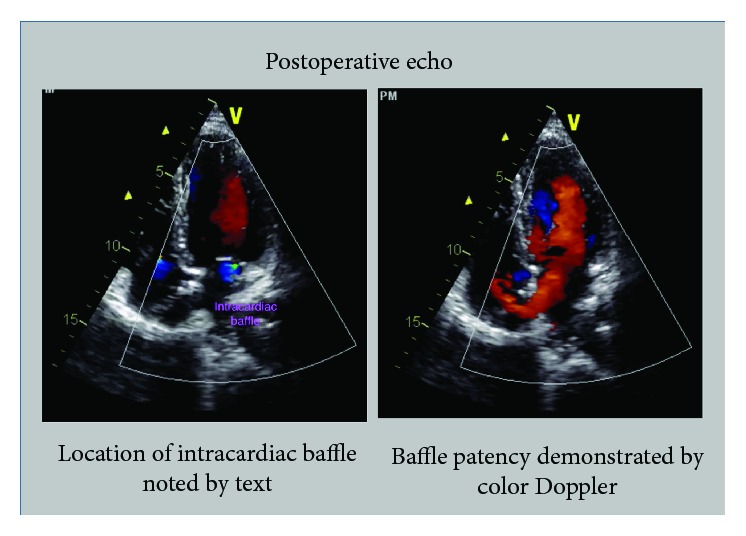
Echocardiogram, apical four chamber view. The left frame shows the baffle traversing the right atrium and opening into the left atrium. The right frame shows color flow through the baffle into the left atrium.
